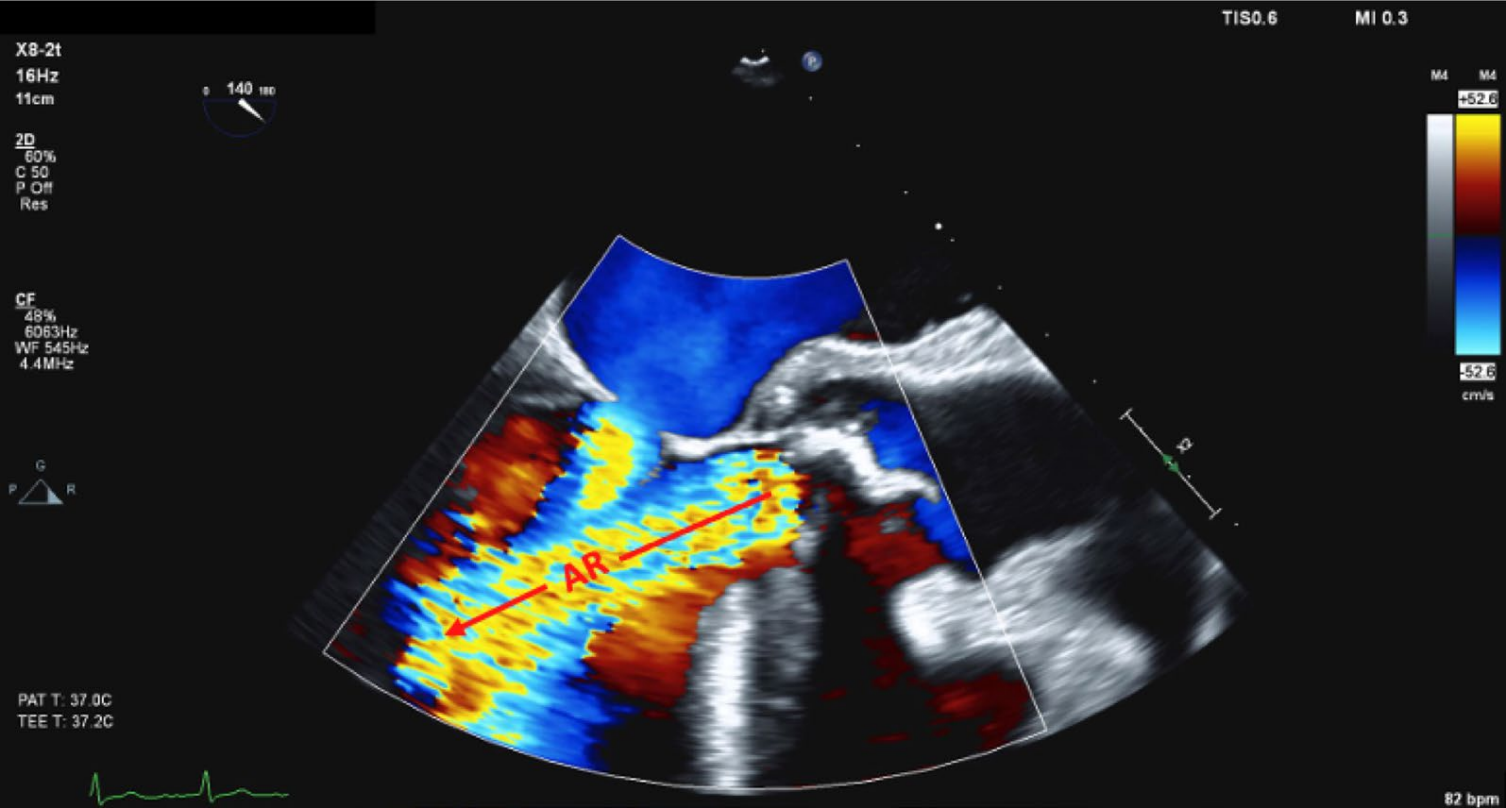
Transesophageal echocardiogram still in mid-esophageal long-axis with an imaging plane of 140 degrees but with color Doppler applied. The labelled structures in image A correlate with the above still. As per convention, the color blue denotes flow away from the probe and red towards. Color grading denotes the velocity of the flow in centimeters per second. Jet of aortic regurgitation is labeled “AR,” with an arrow denoting the direction of flow. Other than the jet of aortic regurgitation, it can also be seen that there is antegrade flow across the mitral valve from the left atrium into the left ventricle, denoting that this image was taken during diastole
